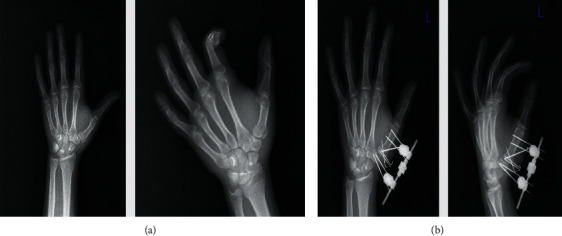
(a) Preop AP and oblique X-ray views of Rolando fracture. (b) Postop AP/lateral X-ray views of Rolando fracture.
